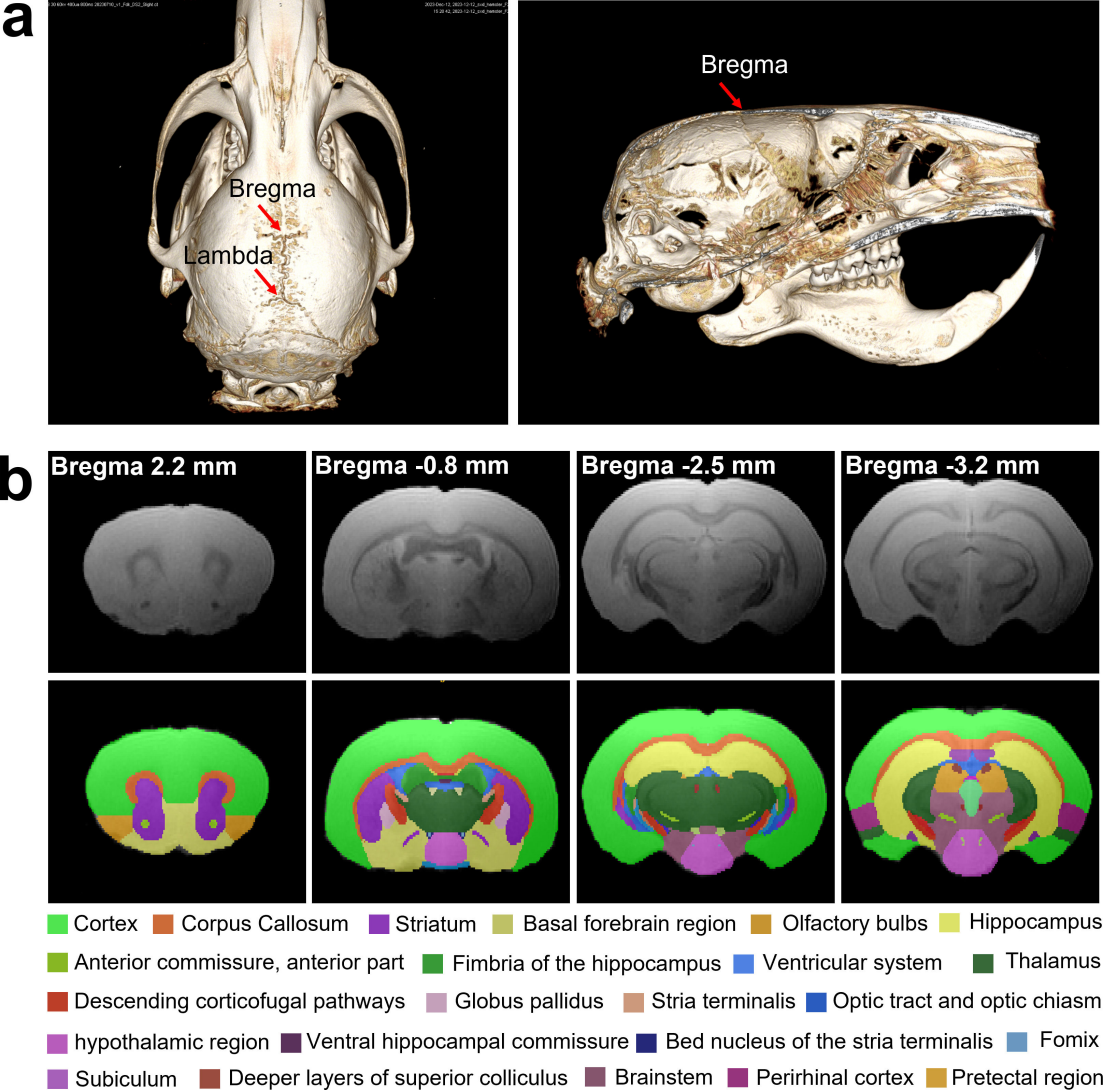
(a) CT images of the head of a hamster with the brain. (b) The labeled MRI template of the hamster brain with the axial plane.
